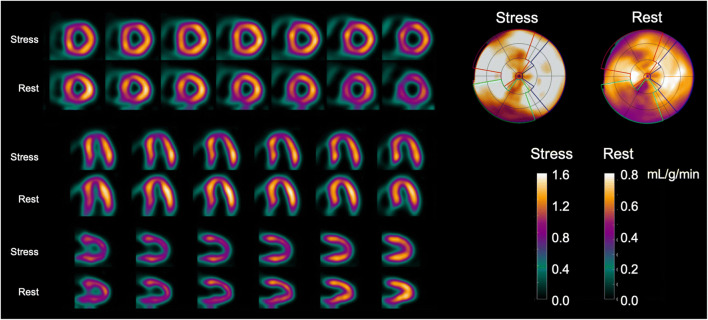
[^15^O]water parametric MBF images from a 65-year-old female with angina referred for assessment of ischaemia with PET. The images shown here are parametric MBF images based on 4-min dynamic [^15^O]water PET scans, with their corresponding polar maps. Note that colour scales of all images represent MBF in mL/g/min as seen in the colour bars. SPECT was negative. PET clearly shows balanced ischaemia with stress MBF far below the threshold of 2.3 mL/g/min
